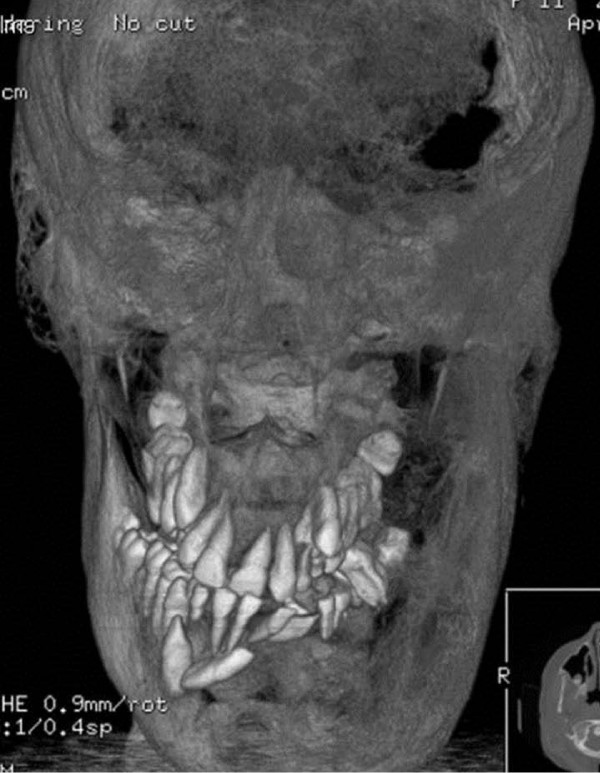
Three-dimensional reconstruction with volume rendering from a computed tomography scan of her skull. Shows facial disfigurement due to extended bone distensions with mixed radiolucent and sclerotic focal lesions.
